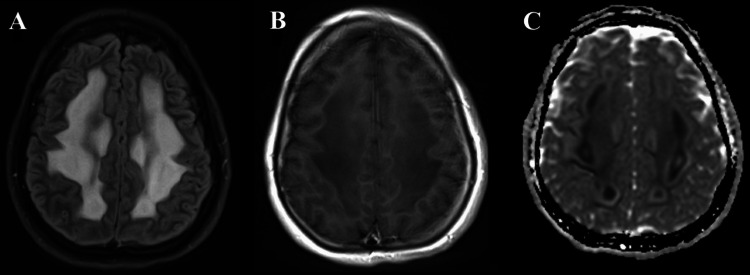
MRI images from the same level demonstrate a high signal on FLAIR (A), without enhancement on the post-contrast T1 images (B). Restricted diffusion is noted throughout the affected areas, as seen on the ADC (C). FLAIR: fluid-attenuated inversion recovery; ADC: apparent diffusion coefficient
